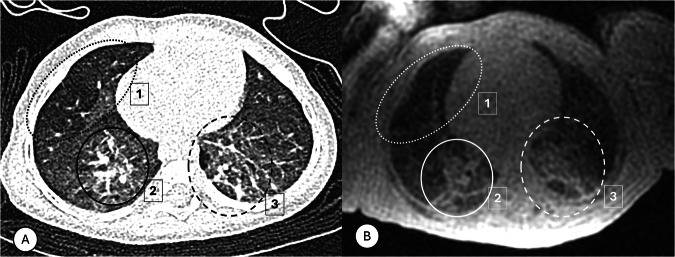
Comparison of A CT and B ZTE MRI sequence at corresponding anatomical levels in a BPD patient. Circle 1 (dotted line) highlights a hypoattenuated region on the CT-scan, which is more difficult to identify on the MRI. Circle 2 (continuous line) shows fibrotic strings visible on both CT and MRI. Circle 3 (dashed line) marks a combination of hypoattenuated regions and fibrotic strings, clearly visible on both imaging modalities. Minimal progression was observed between the two scans, with a slight improvement on the CT. ZTE, Zero echo time; BPD, Bronchopulmonary dysplasia
